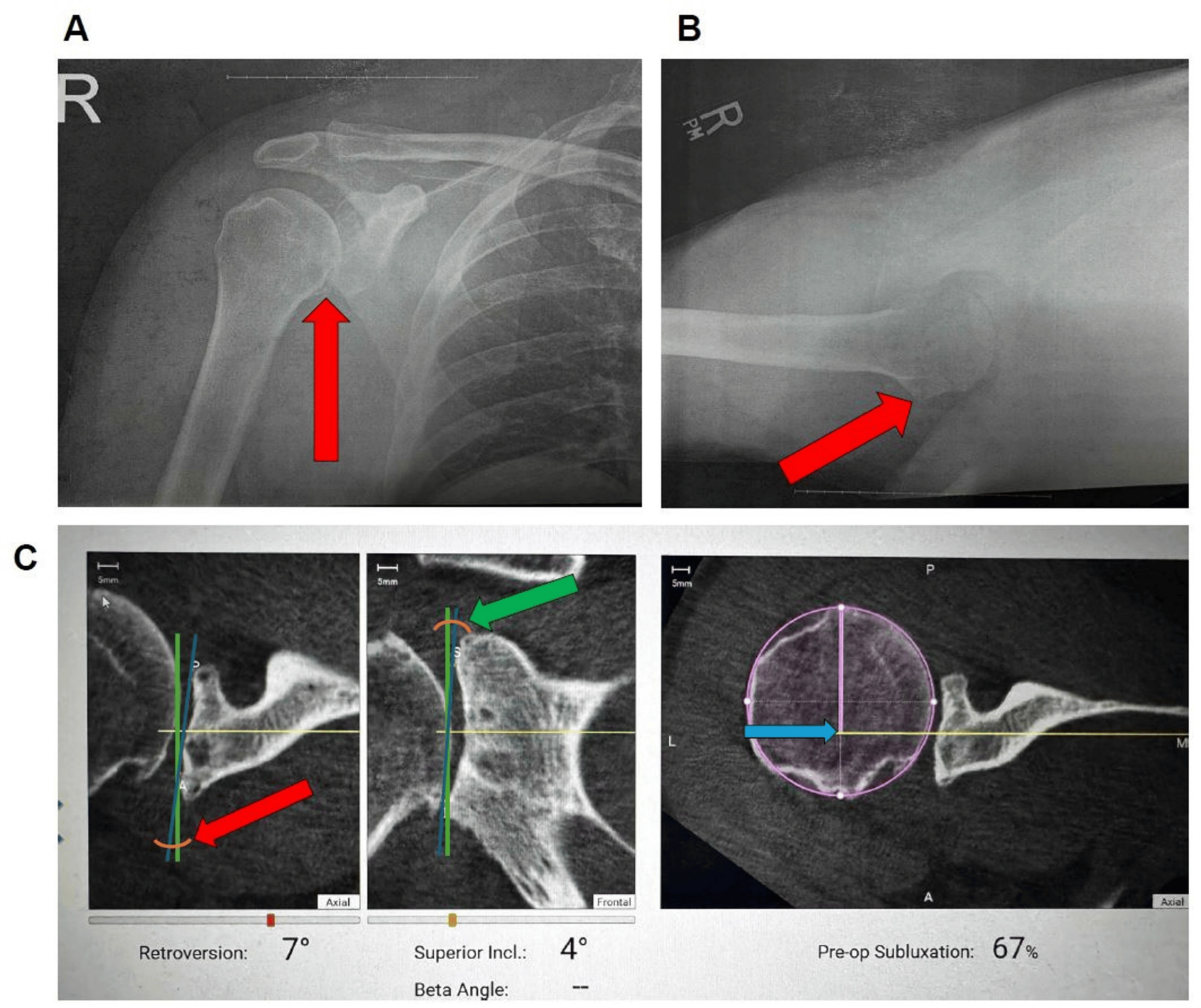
Preoperative imaging and ExactechGPS navigation system (Exactech, Inc., Gainesville, FL) planning for anatomic total shoulder arthroplasty. (A) Anteroposterior radiograph of the right shoulder demonstrating inferior humeral head osteophyte formation with associated subchondral sclerosis, consistent with early glenohumeral osteoarthritis. (B) Axillary radiograph of the right shoulder demonstrating humeral head contour irregularity, consistent with degenerative change. (C) Preoperative GPS planning images quantifying glenoid retroversion of 7° (red arrow), superior inclination of 4° (green arrow), and posterior humeral head subluxation of 67% (blue arrow), consistent with eccentric posterior wear. GPS, global positioning system
